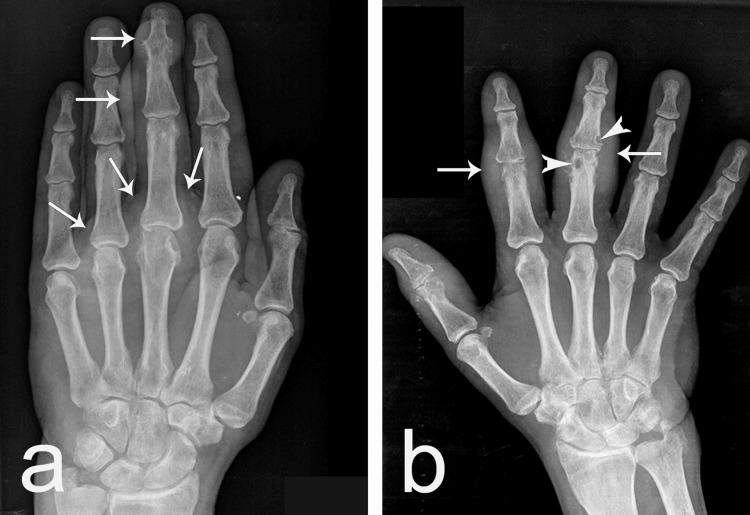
X-rays of both hands of the patient (a) Left hand; (b) right hand; white arrows: gouty tophi presented as soft tissue mass; white arrowheads: bone cysts
